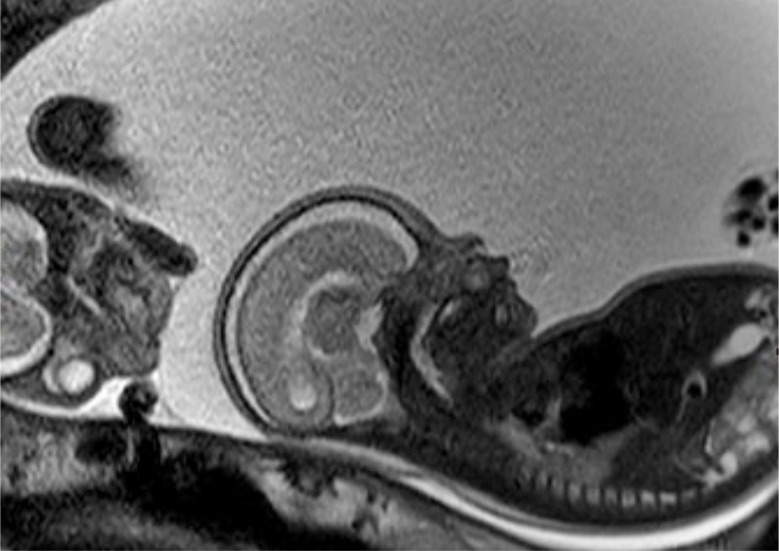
Fetal MRI at 25 weeks of gestation: the fetal MRI picture reveals the massive polyhydramnios. Due to the polyhydramnios of fetus II, fetus I provided a similar picture like a stuck twin, as it is found in a twin-to-twin transfusion syndrome, although fetal development and amniotic fluid have been normal in the second twin.
